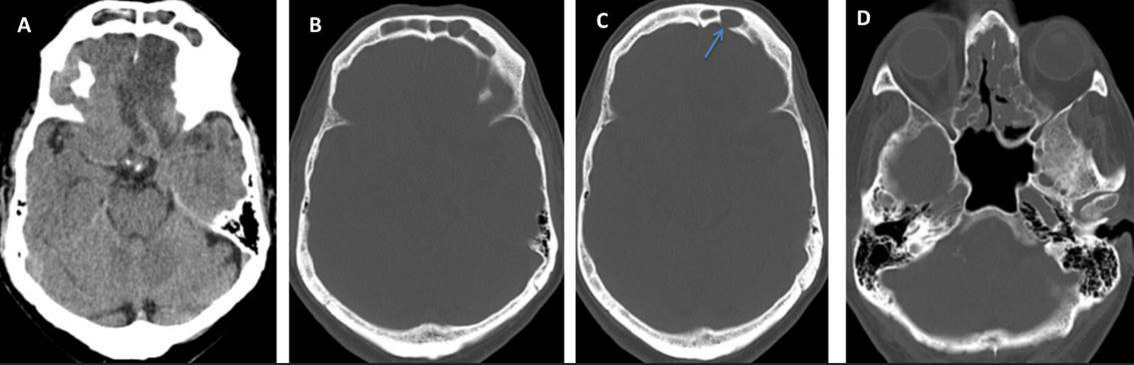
Axial scan cerebral CT in A and B (bone window) shows occupation of both frontal sinuses by soft tissue, which cause erosion of its left posterior wall (arrow in C). These features are compatible with frontal mucocele. In addition, polyposis at bilateral ethmoid sinus was associated.
